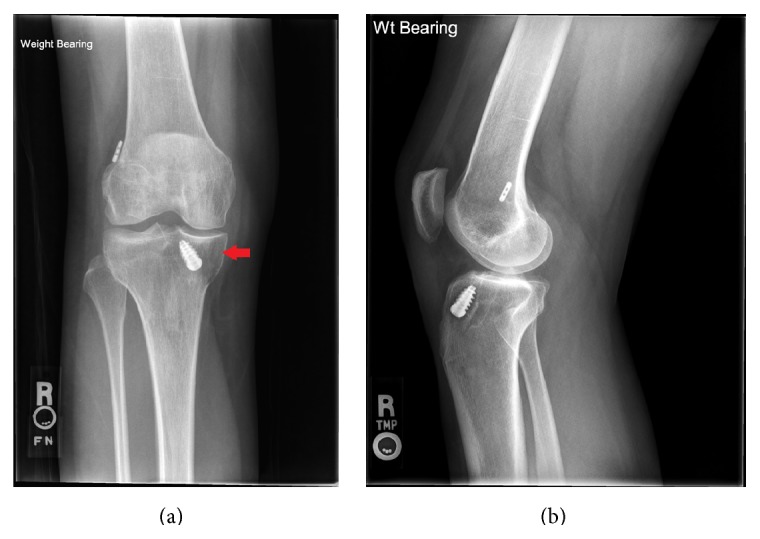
AP (a) and lateral (b) weight bearing radiographs of right knee obtained at the time of knee reinjury, approximately 6 months after the ACL reconstruction surgery. Retrospectively, new lucent lesion adjacent to the tibial tunnel is present (arrow).
